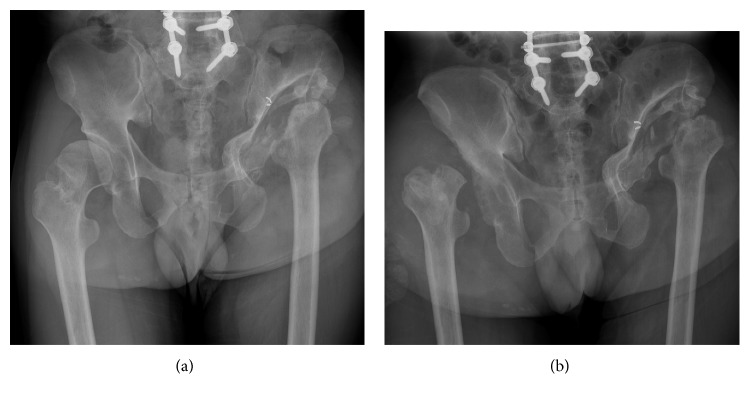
Case 2 preoperative hip X-rays. (a) Two months before admittance to our hospital. (b) Preoperative hip X-ray. A hip X-ray revealed the disappearance of right femoral head and destructive changes in the right acetabulum.
